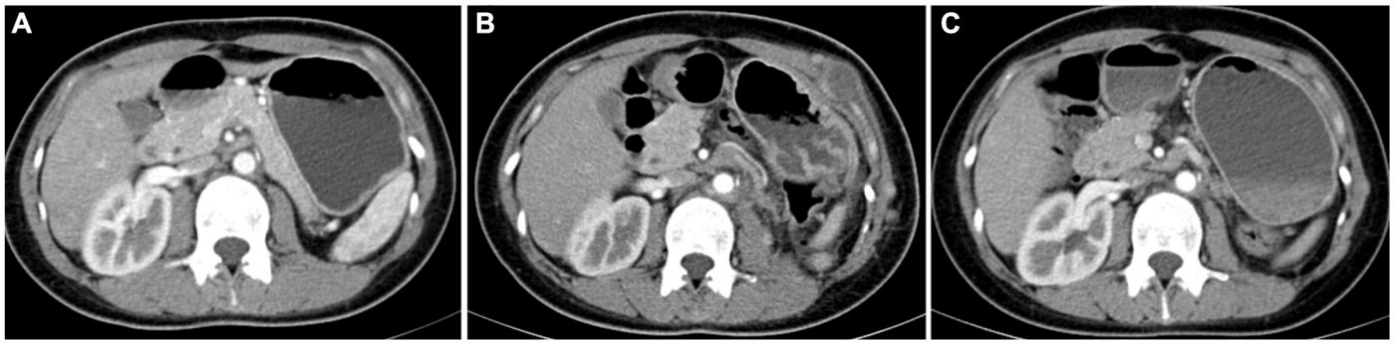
Contrast-enhanced computed tomography results of postoperative follow-up in a patient with TFE3-RCC. (A) The results of re-examination one month after the surgery; (B) three months after surgery, the patient’s re-examination showed multiple tumor metastases in the abdominal wall, left psoas major muscle, and pelvic cavity, so the patient started sunitinib therapy; (C) the re-examination results at six months after surgery showed that the metastasis was smaller than before, and the disease progression was controlled.
